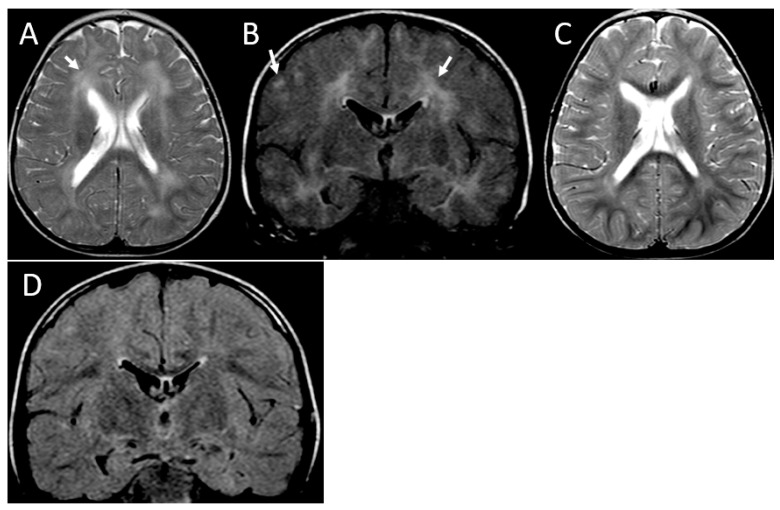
MRI of partially reversible leukoencephalopathy. Patient 3. Initial MRI at the age of 16 months, axial T2-weighted (A) and coronal FLAIR (B) images demonstrate bilateral asymmetric hyperintense areas involving subcortical and periventricular white matter (arrows). Follow-up MRI at the age of 26 months, axial T2-weighted (C), and coronal FLAIR (D) images showing improvement of the abnormal areas.
